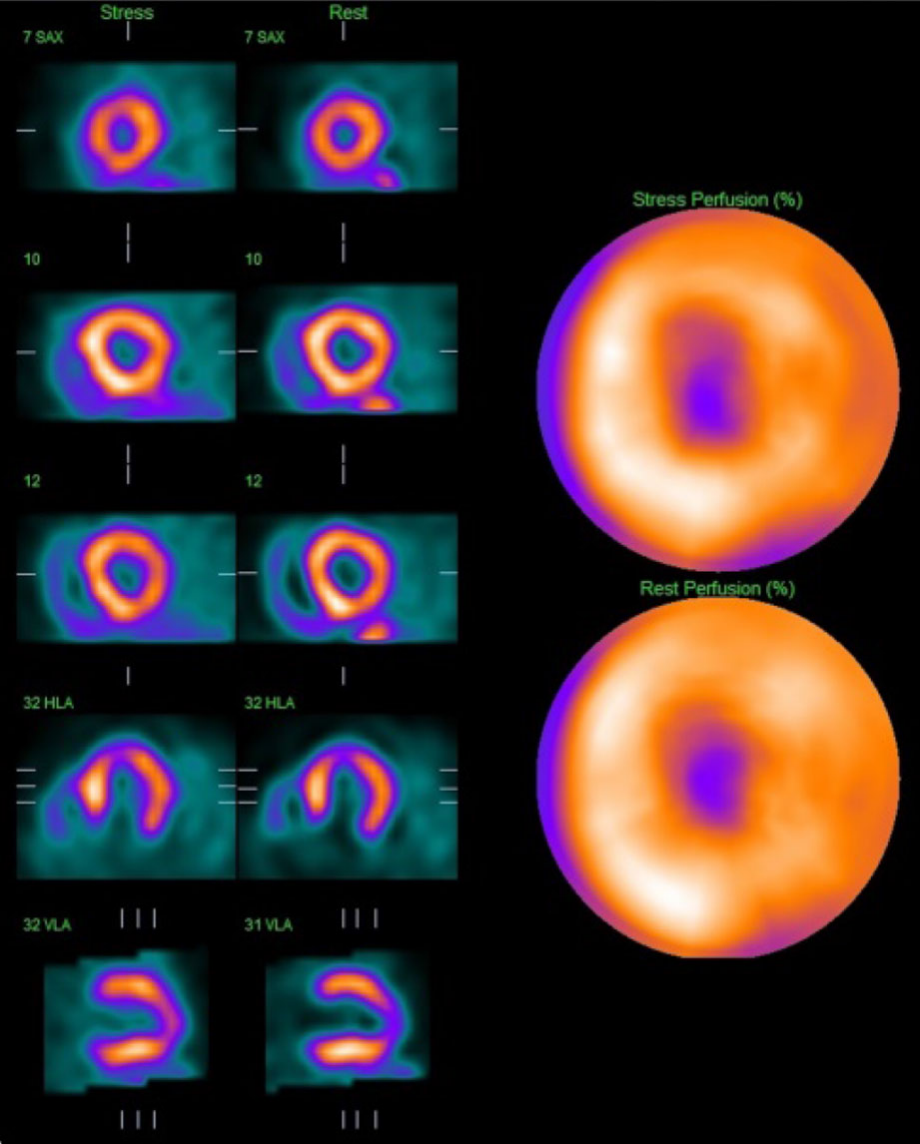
Case 3: Tc-99m tetrofosmin MPS of a 75-year-old woman with ILD and pulmonary hypertension showing mild septal flattening, a partially reversible apical anteroseptal defect and possible (minor) defect in the basal lateral wall. ILD, interstitial lung disease; MPS, myocardial perfusion scintigraphy; Tc, technetium
